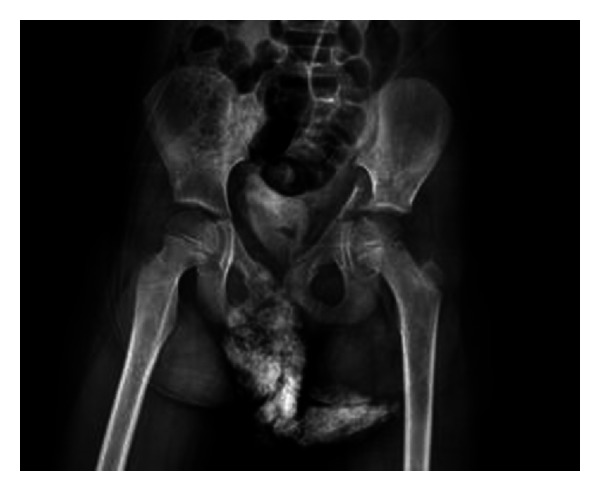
Preoperative pelvis AP X-ray.
